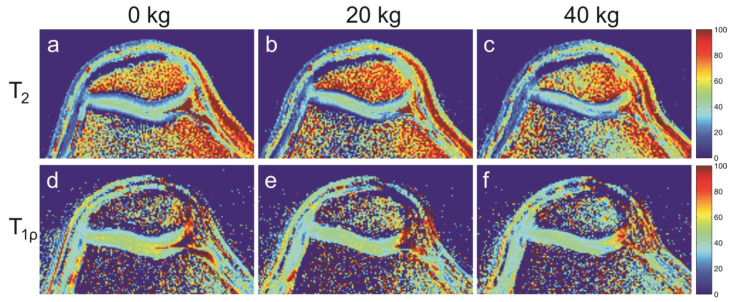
Color-coded T_2_ and T_1ρ_ maps. Shown are color-coded T_2_ (a–c) and T_1ρ_ (d–f) maps (in ms) of the patellofemoral cartilage from MRI measurements on a healthy subject performed with in situ loads of 0, 20, and 40 kg. This figure is a reprint of Figure 4, originally published in [122]. Permission for this reprint has been obtained.
